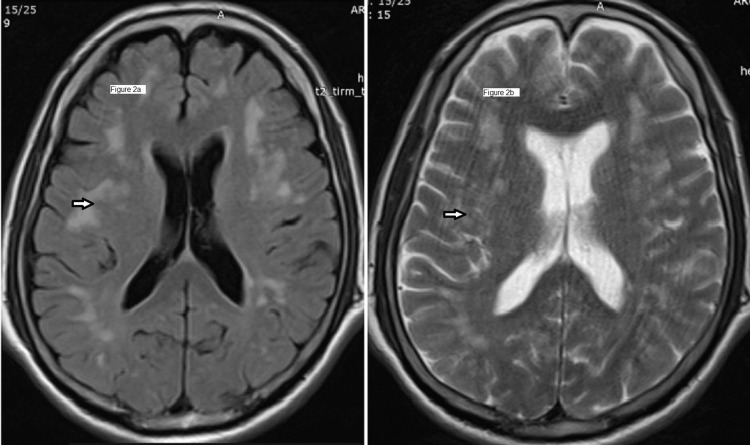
MRI brain showing multiple patchy and confluent areas of hyperintensities in subcortical and periventricular white matter of bilateral cerebral hemispheres in T2-weighted image (T2-WI) and fluid attenuation inversion recovery (FLAIR) image. Figure 2a is MRI brain T2-weighted image (T2-WI) and Figure 2b is MRI brain fluid attenuation inversion recovery (FLAIR) image, both showing patchy and confluent areas of hyperintensities in subcortical and periventricular white matter of bilateral hemispheres.
